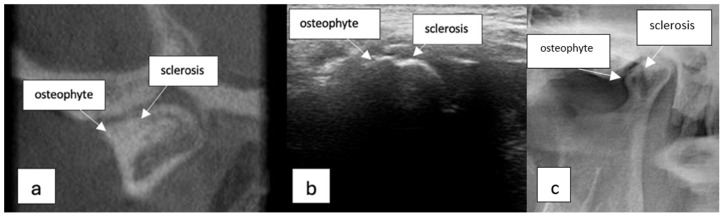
TMJ osteophyte and sclerosis. (a) CBCT image (sagittal view), (b) USG image, and (c) panoramic radiography image.
